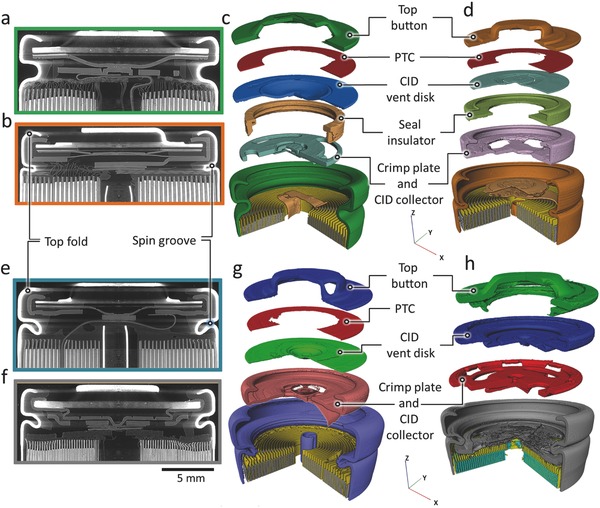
Greyscale *XZ* orthoslices from X‐ray CT reconstructions of a) LG‐S3 and b) Panasonic cells. Exploded 3D reconstructions of c) LG‐S3 and d) Panasonic cells, showing the placement of the integrated safety devices. Greyscale *XZ* orthoslices from e) Sanyo and f) Samsung cells. Exploded 3D reconstructions of g) Sanyo and h) Samsung cells, showing the placement of the integrated safety devices.
